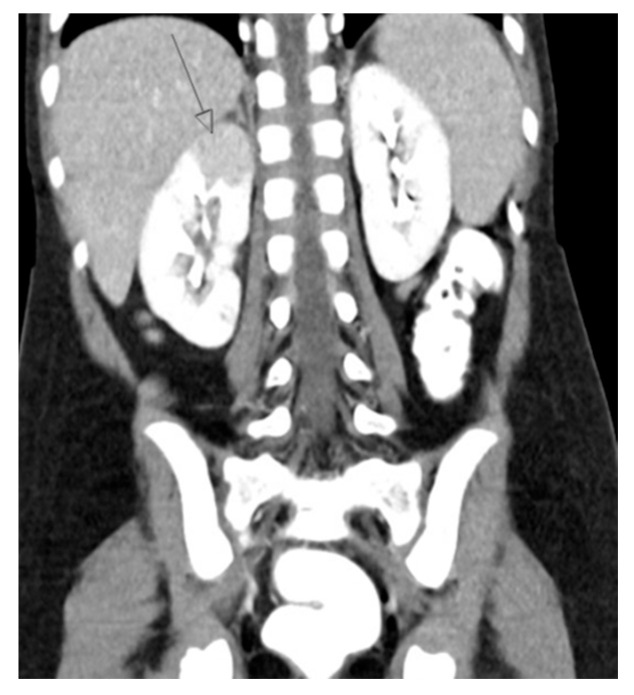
CT shows right sided pyelonephritis (indicated by the arrow) in a 4-year-old female.
